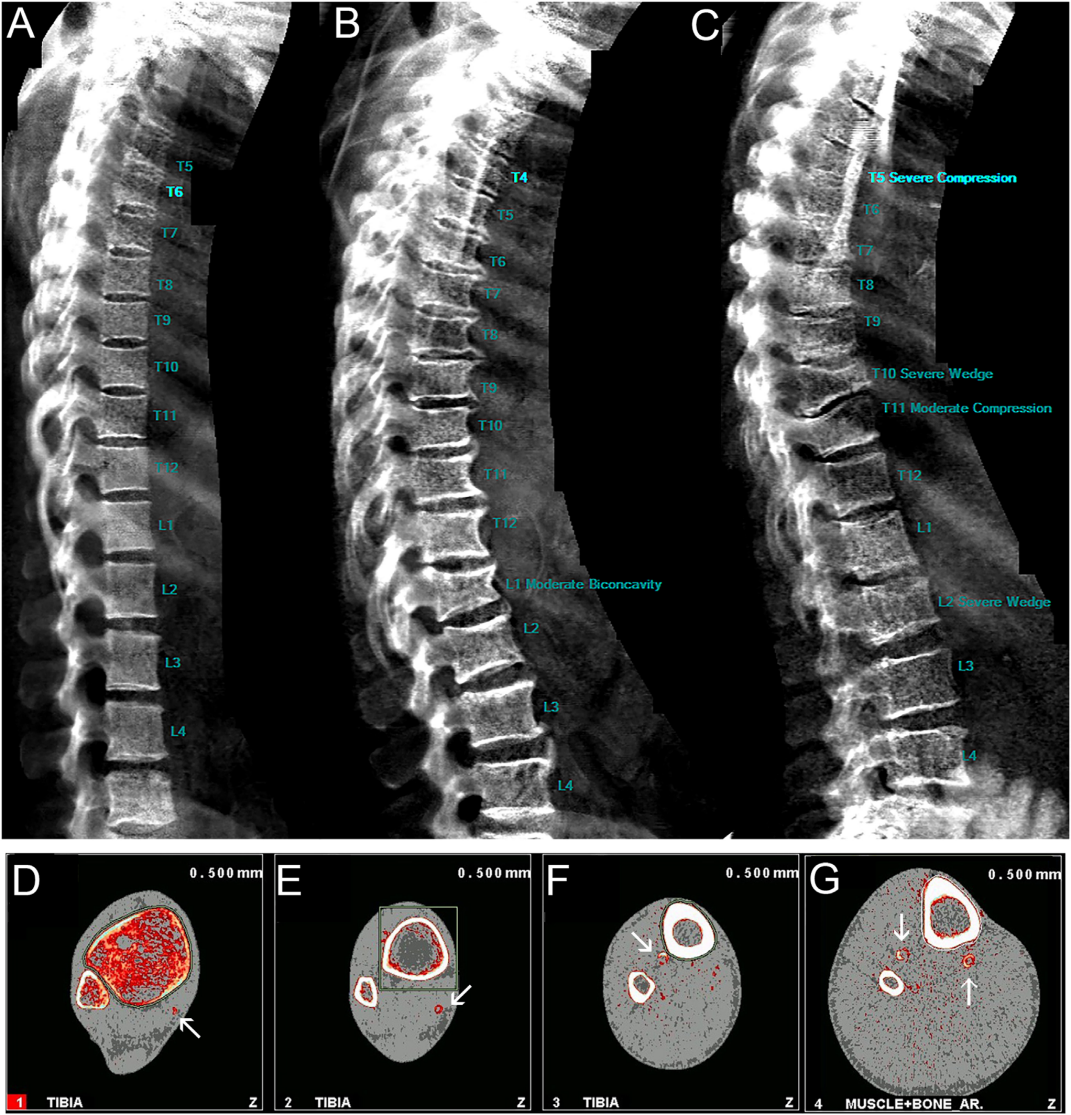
Representative lateral vertebral assessment scans of (A) normal vertebrae, (B) biconcavity at the spine, (C) severe compression and wedge fractures; representative peripheral quantitative computed tomography scans with *white* arrows indicating vascular calcification from the (D) 4%, (E) 14%, (F) 38%, and (G) 66% tibia.
